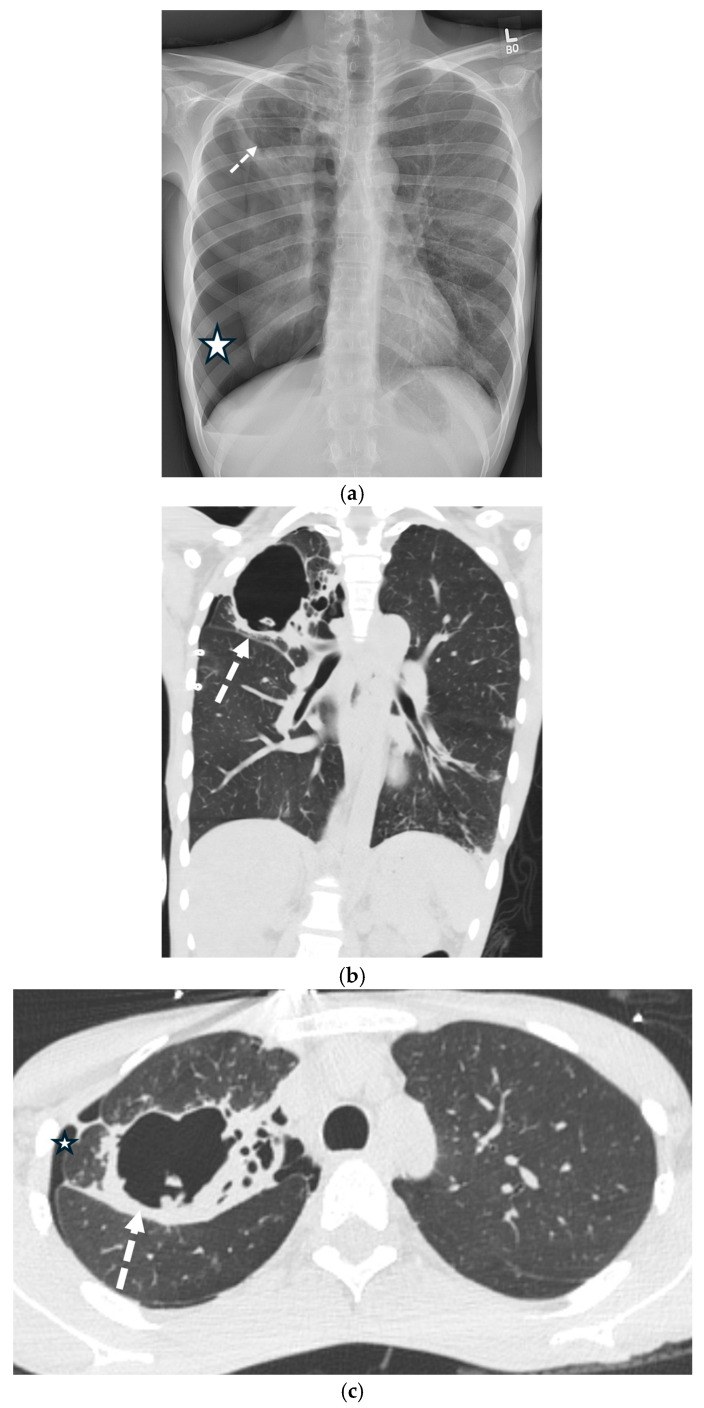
34 -year-old male presents with right chest pain. Frontal chest radiograph (a) demonstrates moderate-to-large right pneumothorax (large white star) and right-upper-lobe thick-walled cavitary lesion (white dashed arrow). Coronal and axial CT images in lung window (b,c) demonstrate small right pneumothorax (small star) status post-right chest tube placement. Note a large thick-walled cavitary lesion (white dashed arrow) in the right upper lobe with adjacent bronchiectasis. Sputum culture confirmed mycobacterium tuberculosis.
